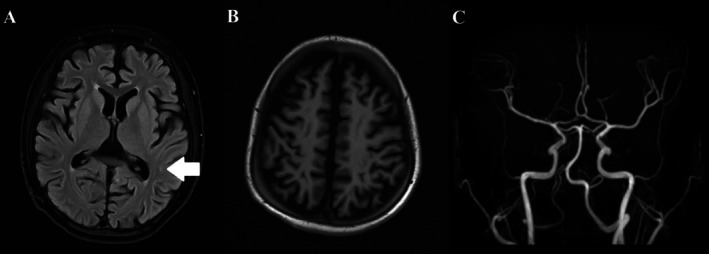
Brain MRI and MRA findings. Image A (T2‐FLAIR): Subcortical T2‐flair hyperintensity. Image B (T1‐weighted): Demonstrates cerebral atrophy. Image C (MRA): No abnormalities observed in the intracranial vasculature.Full spine MRI (Figure 2):FIGURE 2 acn370436-fig-0002:
